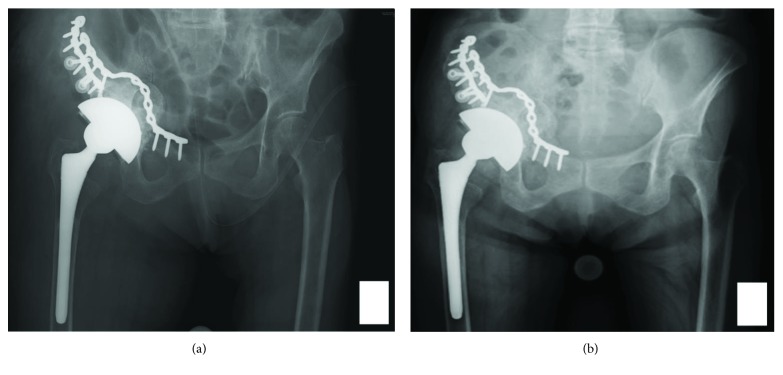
Postoperative radiograph at immediately after surgery (a) and at final follow-up (b).
